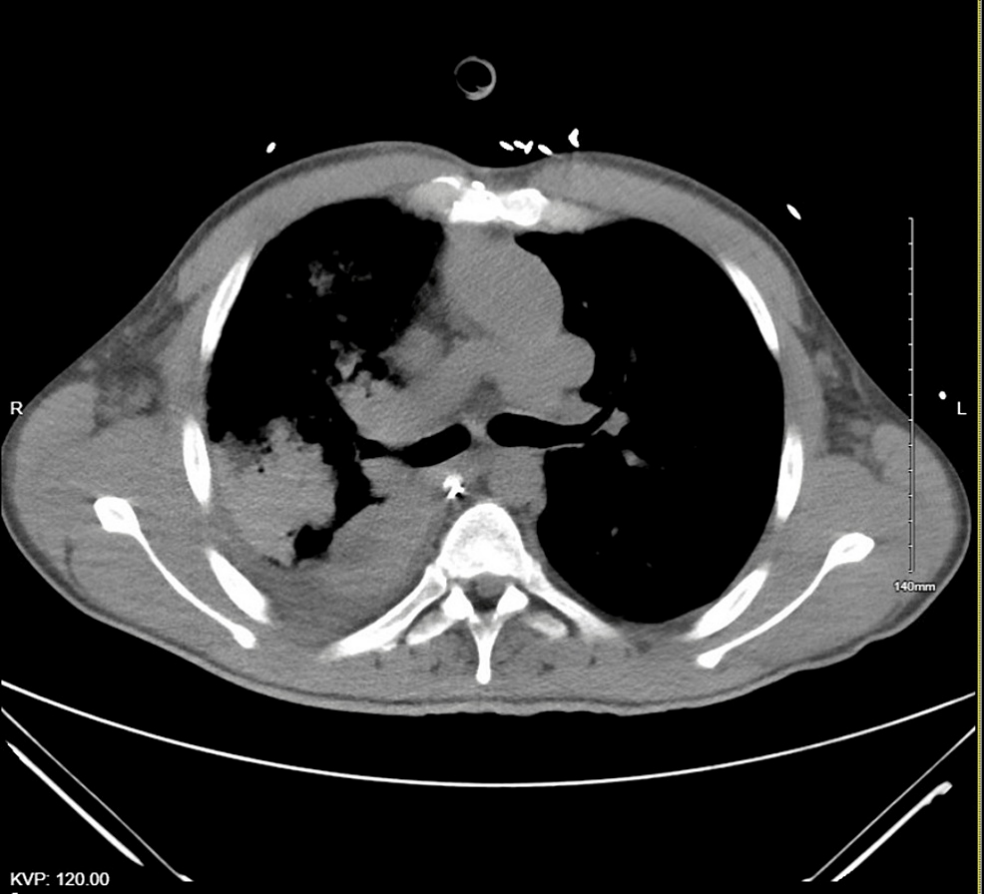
CTPE showing dextrocardia and post-Fontan procedure with the single right ventricle CTPE, computed tomography for pulmonary embolism
